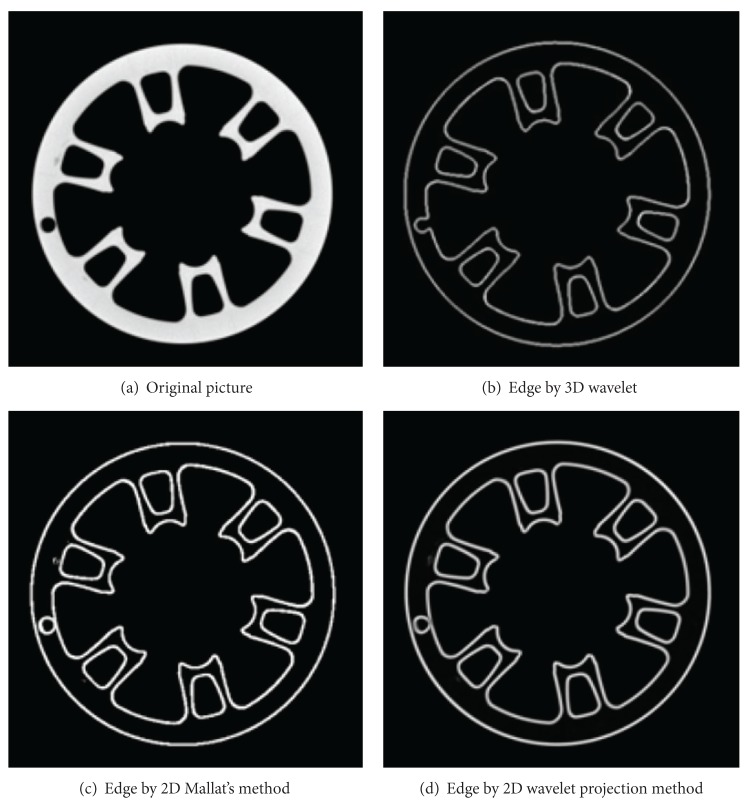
One of 75 wheel hub's CT slices and its edge picture.
